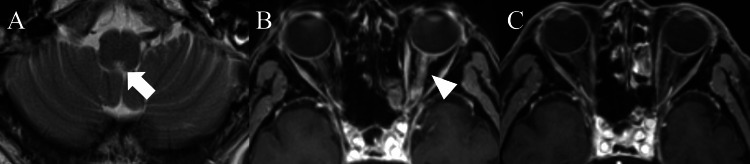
Brain magnetic resonance imaging. The brain MRI showed a slightly hyperintense lesion in the area postrema on day 25 (A, arrow). Contrast-enhanced brain MRI demonstrated enhancement of the left optic nerve on day 64 (B, arrowhead), which improved after immunotherapy (C).
